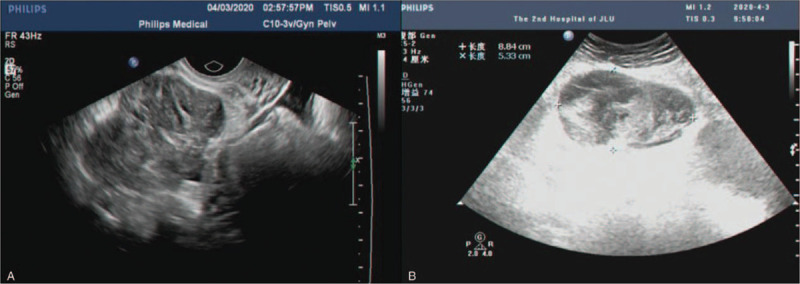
Abdominal ultrasound results of case 1. (A) The ultrasound suggested a 5.9 cm × 4.2 cm heterogeneous hypoechoic between the uterus's anterior wall and the posterior wall of the bladder. (B) The ultrasound indicated multiple hypoechoic nodules in abdominal. Moreover, a big mass was found below the periumbilical abdominal wall.
